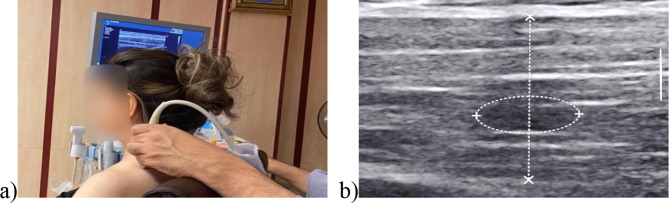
(a) participants position during sonography; (b) TrP CIR in UT muscle gray scale image
